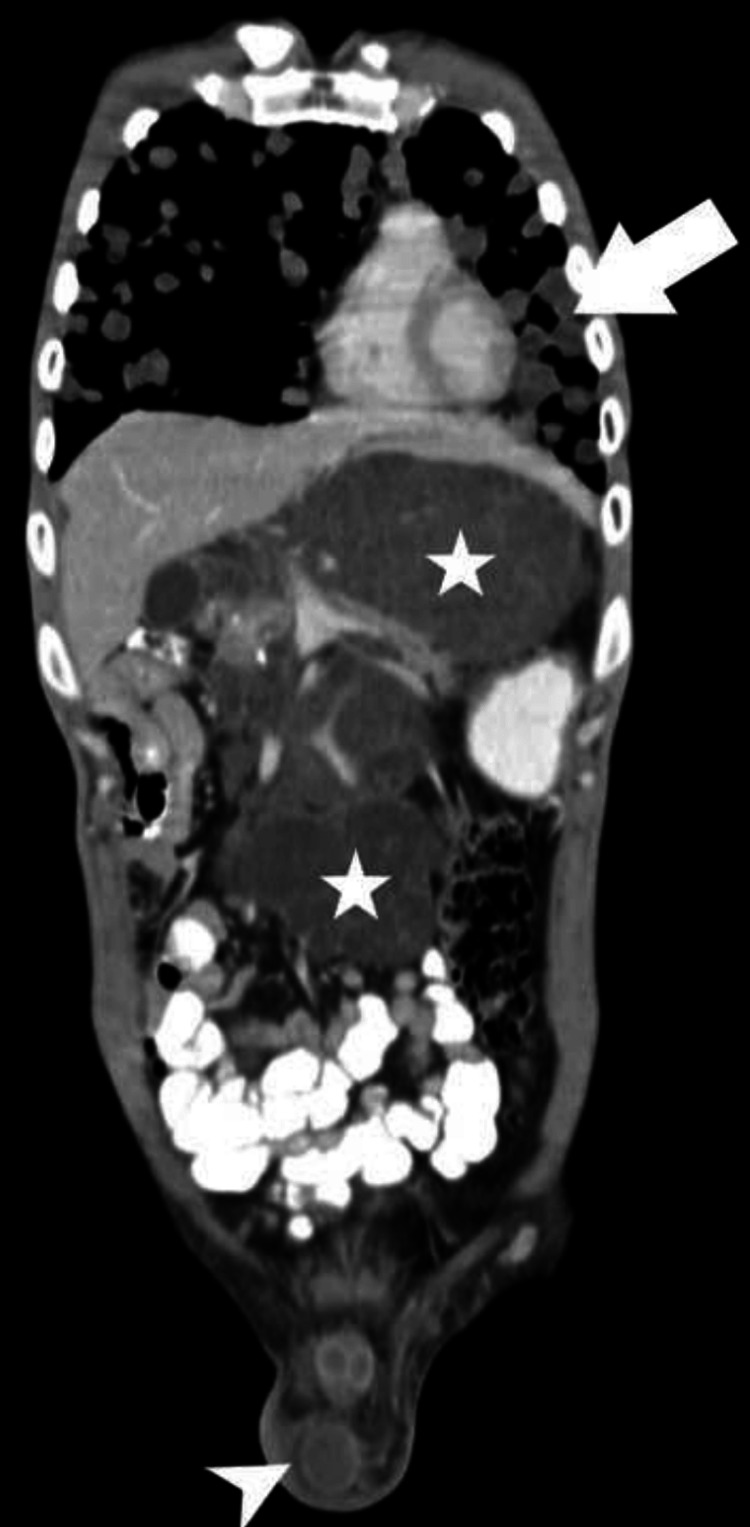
CT mediastinal window showing multiple variable-sized cannon ball metastasis (arrow) in bilateral lung parenchyma. Contrast CT abdomen venous phase showing enhancing lesion (star) in segment VII of liver measuring 5.2 x 4.3 cm and ill-defined retroperitoneal mass (arrowhead) CT: computed tomography
